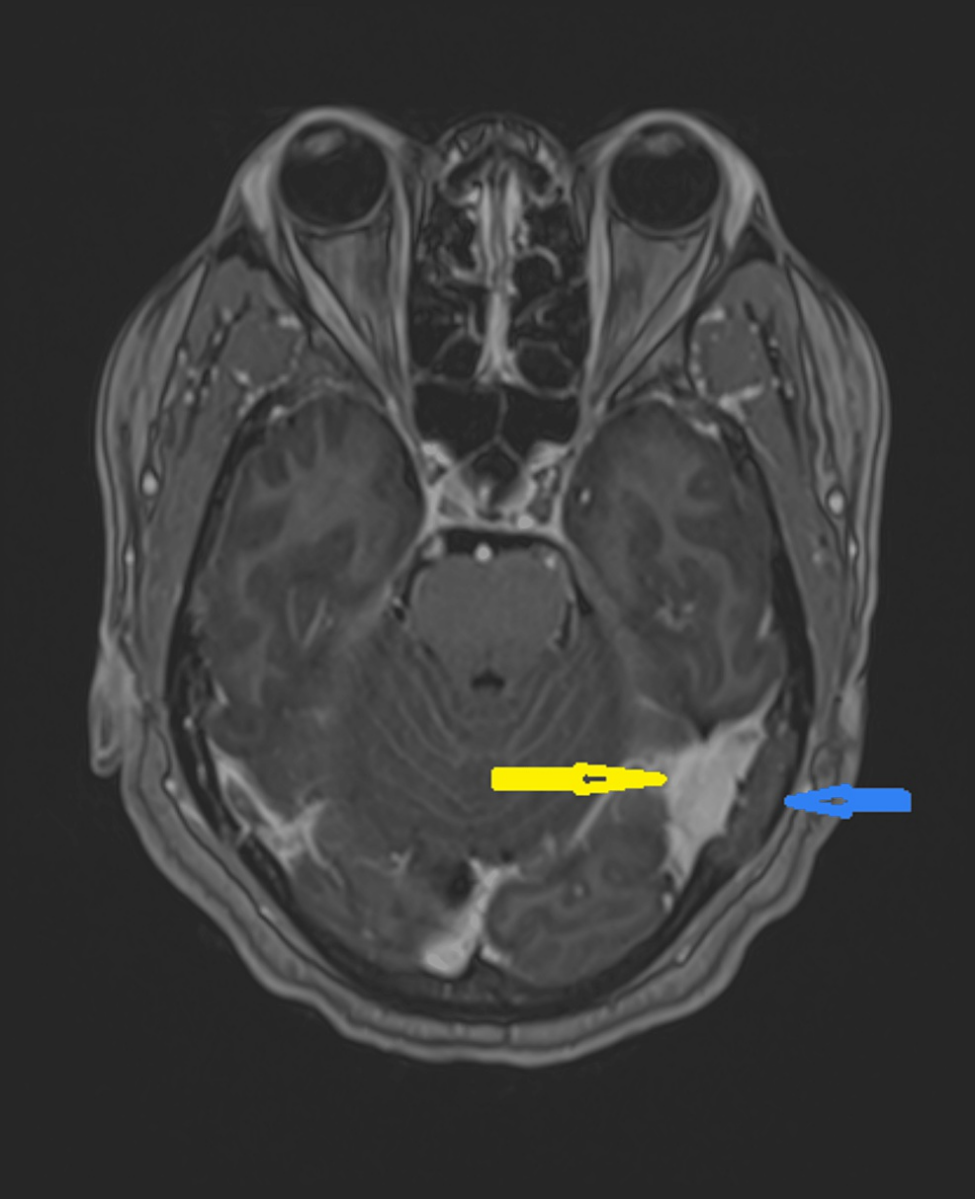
Follow-up MRI head with contrast enhancement Left temporal meningioma en plague (yellow arrow) and hyperostotic growth (blue arrow)
